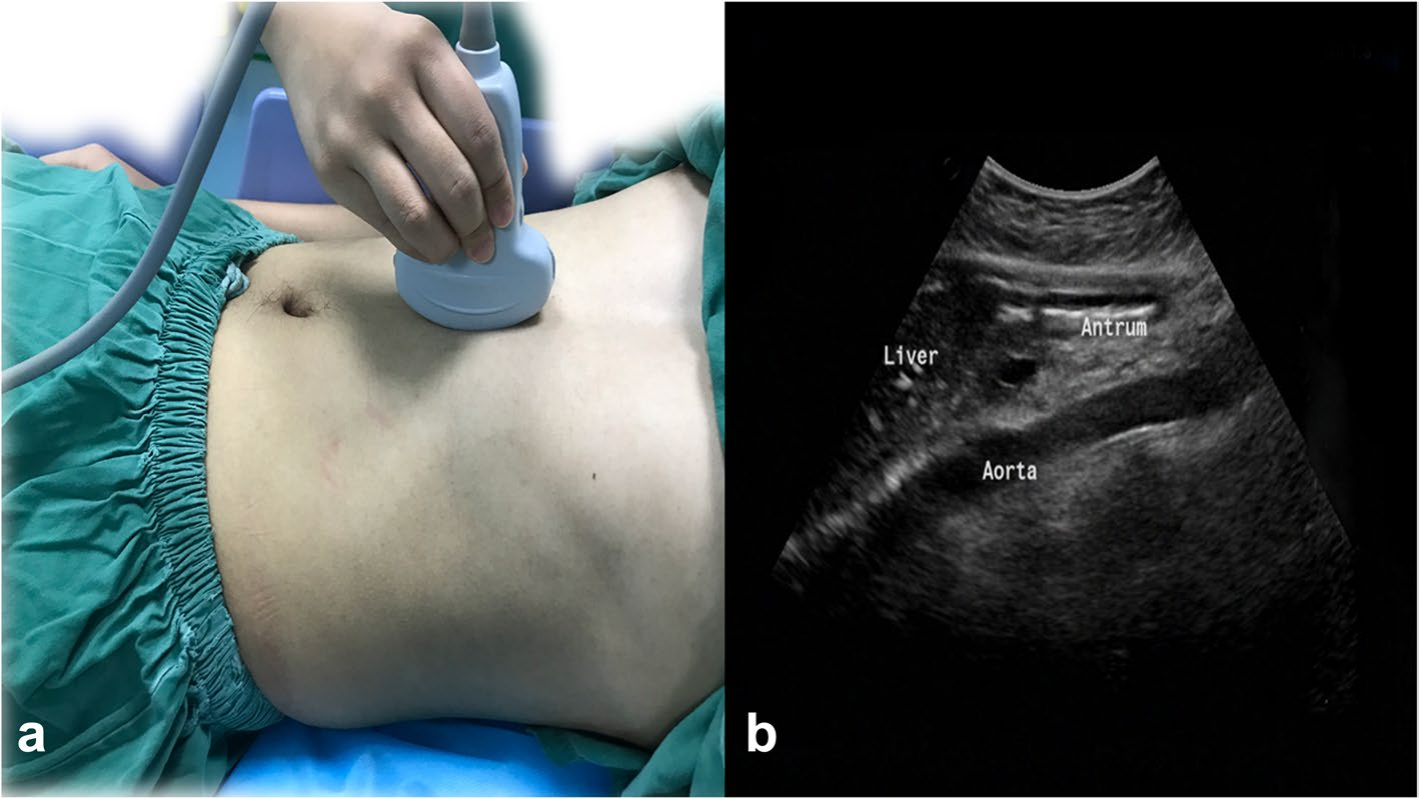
Transducer position to scan the gastric antrum (a). Antral ultrasound image (b)
